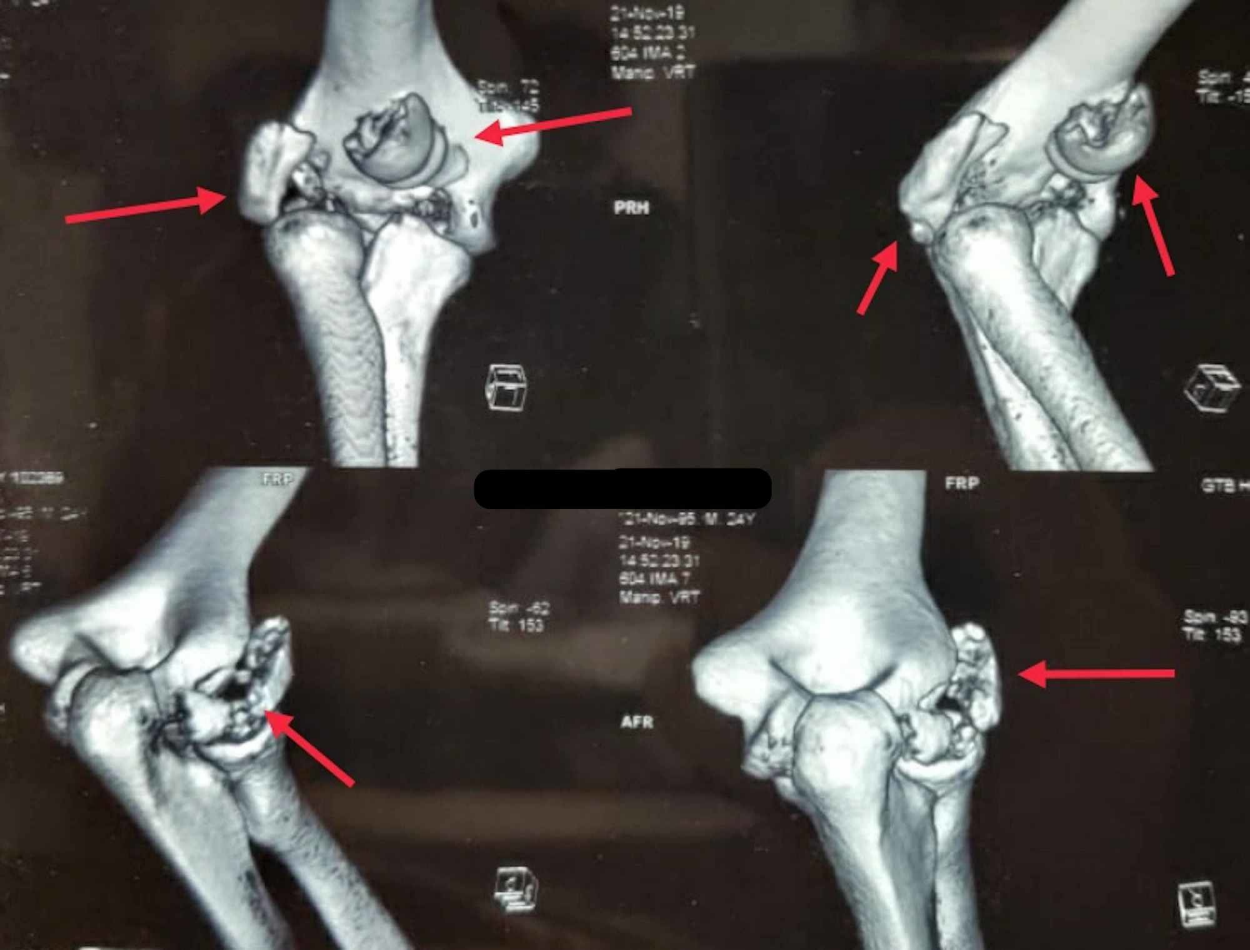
Three-dimensional reconstructed CT images of the right elbow. The arrows denote the capitellar fragments showing the exact fracture anatomy and comminution.
